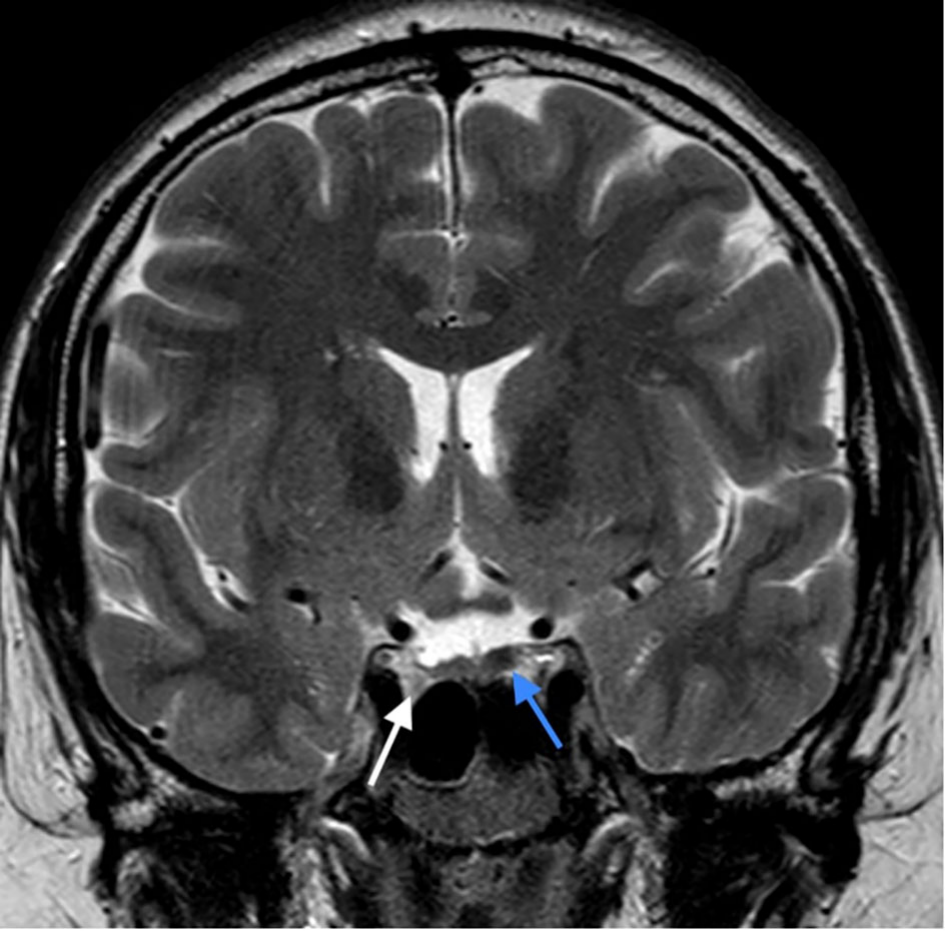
The latest MRI assessment 3 years after initiating the cabergoline treatment: Control T2 weighted image in coronal plane: Size reduction of the right lobe adenoma (white arrow) and almost complete disappearance of the lesion in the left lobe (blue arrow).
